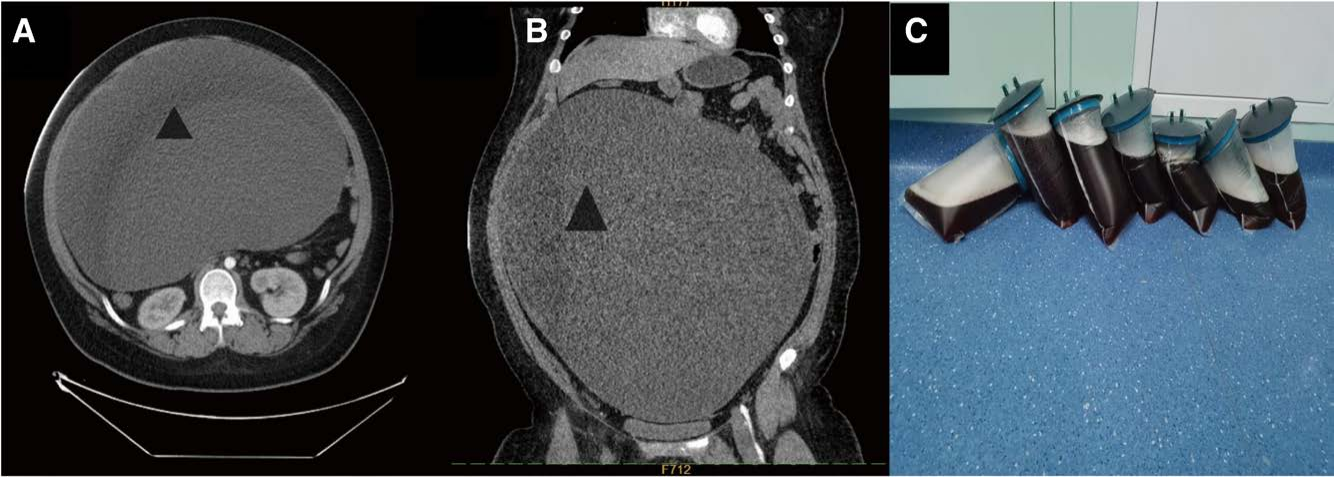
(A and B) Abdominal enhanced CT showing large abdominal tumors with triangles. Figure (C) is the sac fluid extracted from the giant ovarian cystic tumor intraoperatively. CT = computer tomography.
